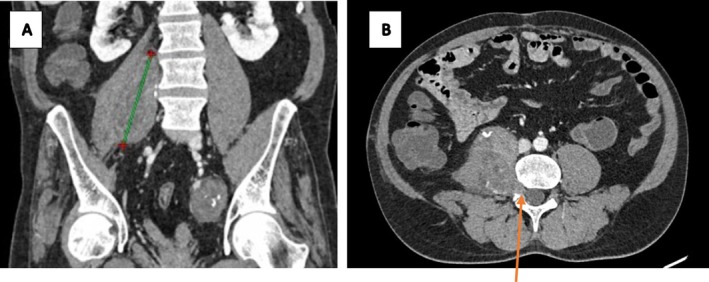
Contrasted CT scan of the abdomen, coronal reconstruction (A) and axial slice (B) showing an ovoid well‐demarcated heterogeneously enhancing mass in the right retro‐peritoneal region, laterally displacing the right psoas muscle, which appears atrophic as compared to the contralateral side. This mass has a connection to a lumbar neuroforamen showing the ‘dumb‐bell’ appearance (orange arrow in the axial image).
